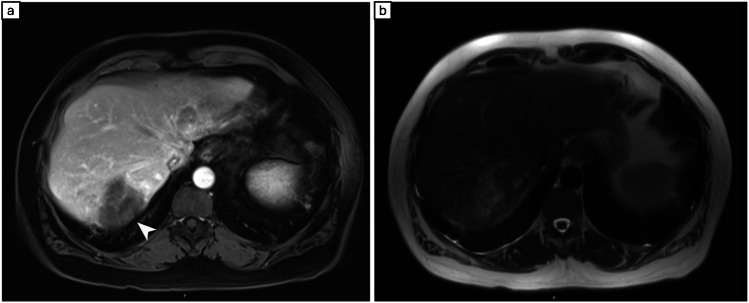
Irregular mass in hepatic segment 7 with hypointensity on T1 sequence (a) and central heterogeneous hyperintensity on T2-weighted images (b) with no evidence of restricted diffusion by magnetic resonance imaging (MRI)
